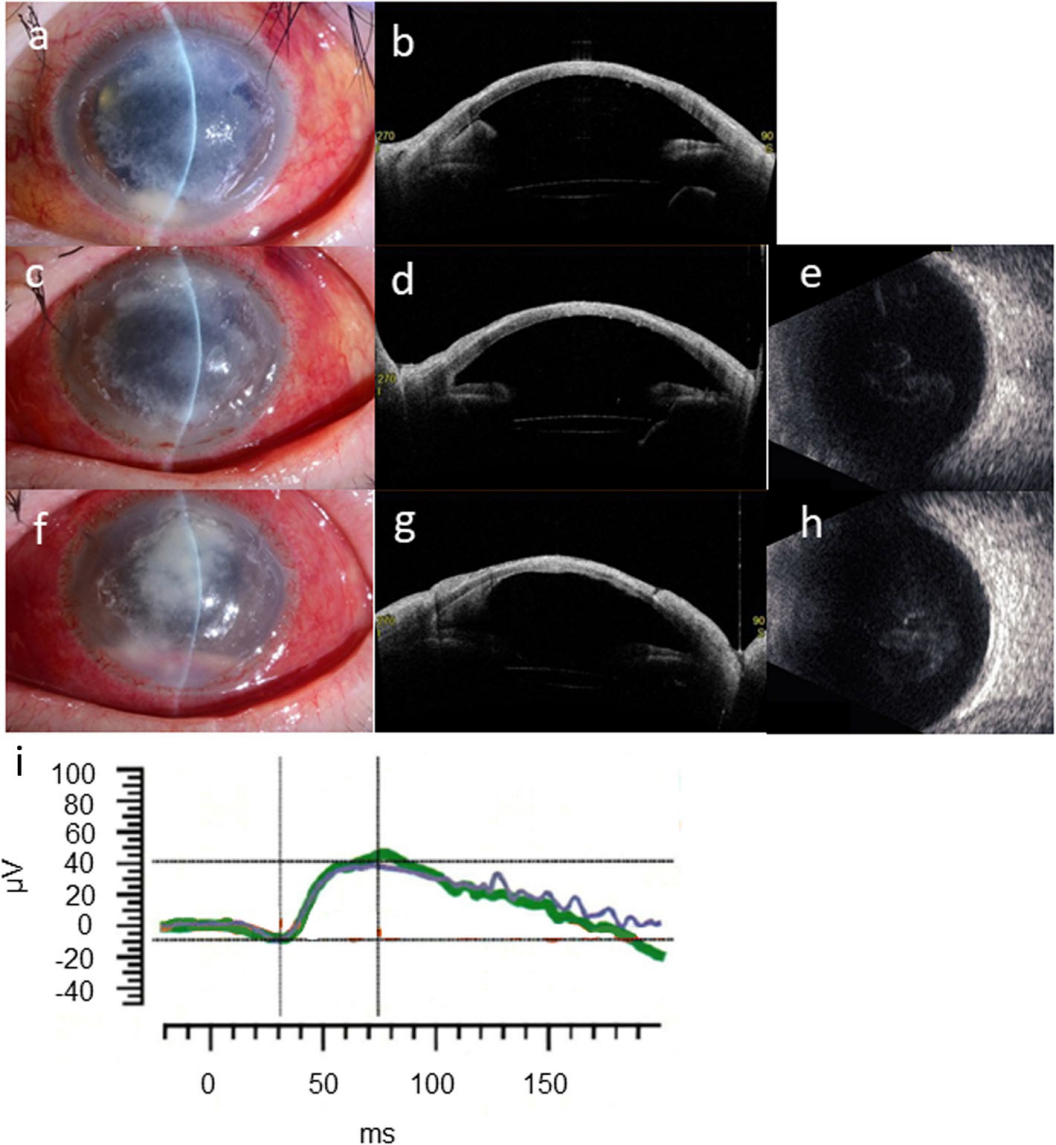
a The corneal infiltrate shrank and ciliary hyperemia improved 2 days after the onset of anti-fungal therapy. b Anterior segment OCT demonstrated decreased hypopyon and anterior chamber fibrin deposition. c and d The day after intravenous tocilizumab injection, aggravation of ciliary hyperemia was noted, although the hypopyon decreased due to anterior chamber irrigation. e Vitreous opacity emerged on echography 2 days after tocilizumab infusion. f and g Slit-lamp examination and anterior segment OCT 10 days after tocilizumab infusion. The range of corneal infiltration increased and the hypopyon worsened. h Hyperechogenic foci on B-scan ultrasonography also worsened. i Reduction of both a-wave and b-wave amplitude was confirmed on full-field ERG 9 days after tocilizumab administration
